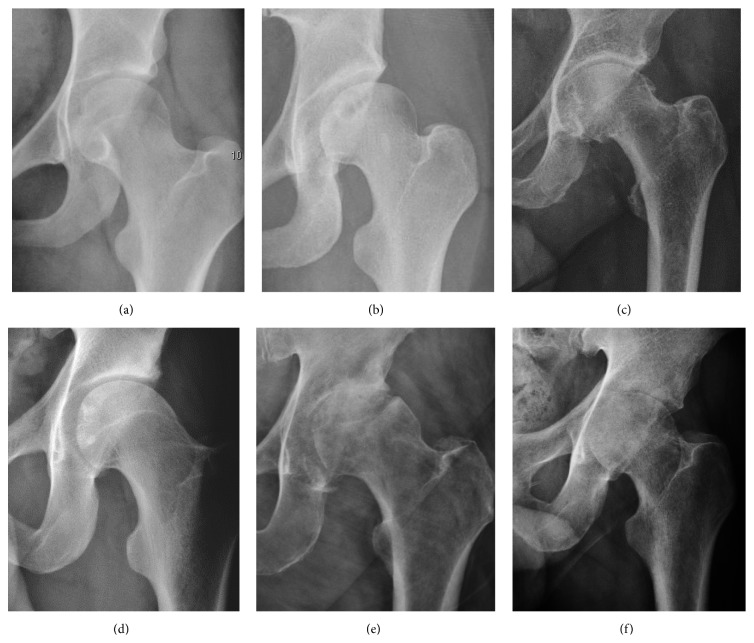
Radiography in normal hip (a), hip dysplasia (b), pincer impingement type (c), and cam (d). Hip in osteoarthritis (e) and septic arthritis (f).
